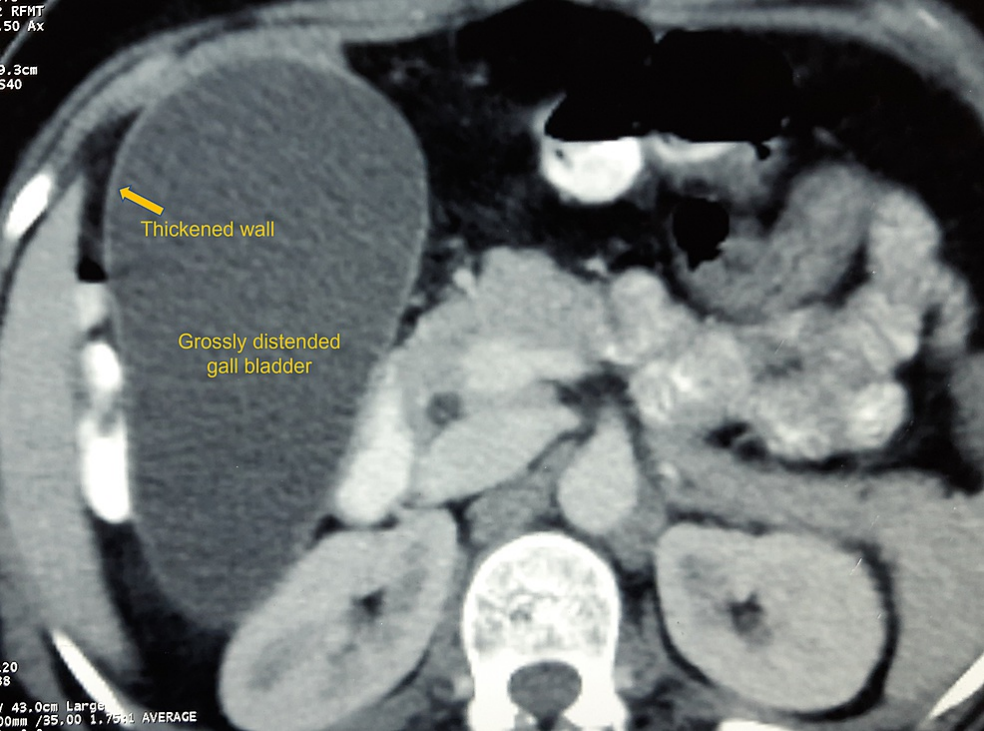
Abdominal computed tomography scan
